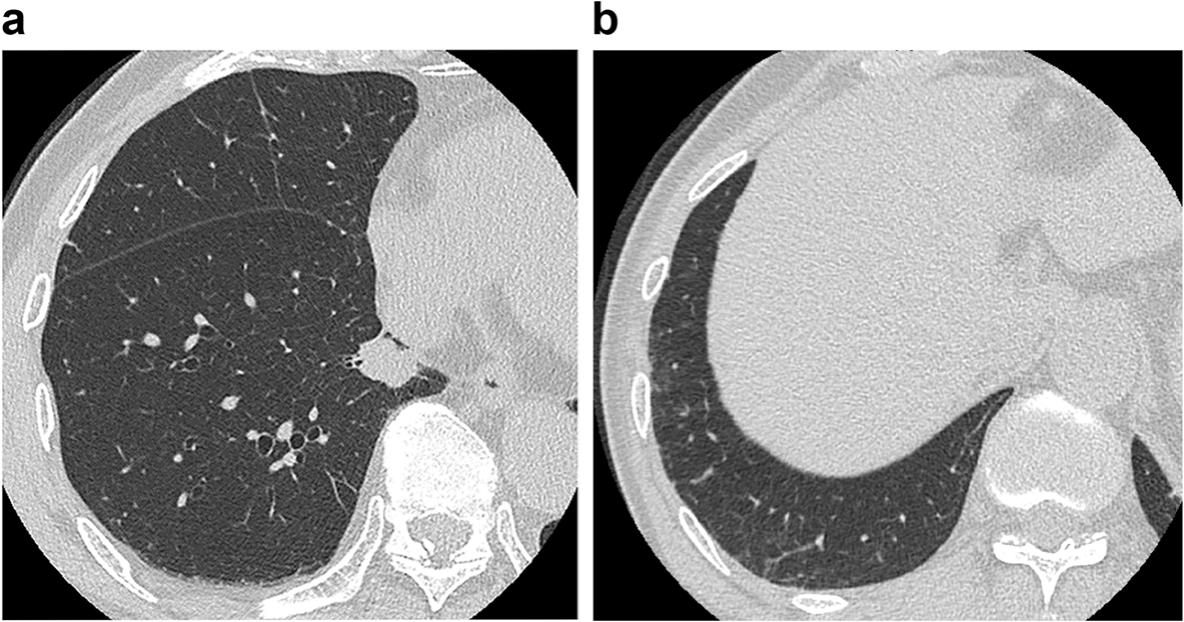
Lung cancer in the normal lung (apart from reticular shadows or emphysema). a The example of computed tomography findings in the lung of a patient with lung cancer. b This is an image showing rest of the lung of patient with fibrosis in Fig. 3a. The image shows mild fibrosis in the right lower lobe; however, it is apart from the lung cancer
